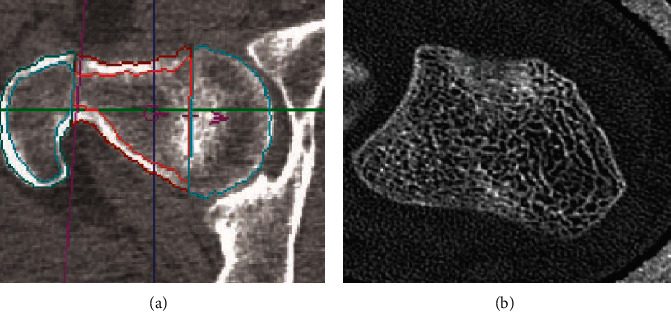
(a) Femora undergo vQCT to determine BMD and macrostructure. (b) Ultradistal forearm undergoes CT to measure the structure of the trabecular complex network and its texture.
